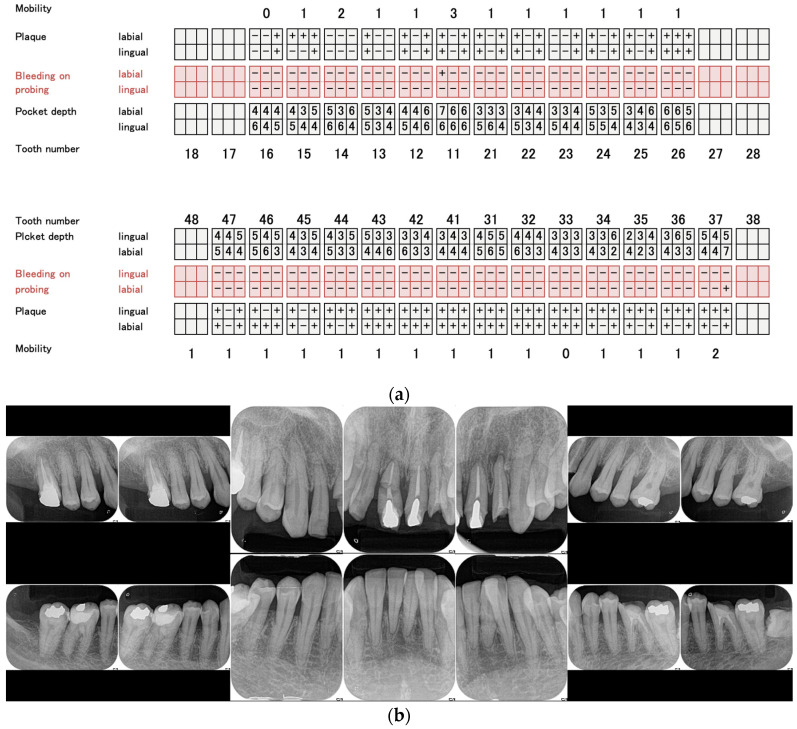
Results of initial periodontal examination (a) and dental radiographs (b).
